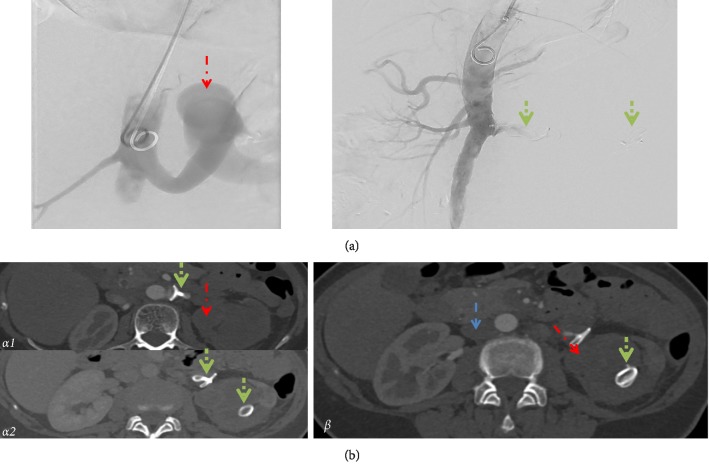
Representative images of an angiography during surgery and a CT scan one-month post-surgery. (a) Angiography before and after Amplatzer Vascular Plug (AVP) II Device placement. No perfusion of the left renal artery (LRA) after AVP II placement. Red arrow (dashed and dotted) = RVA; Green arrow (dotted) = correct positioned central and displaced peripheral AVP II. (b) *α*: arterial phase images. *α*1: no perfusion of the LRA distal of the central AVP II. *α*2: minimal residual cortical perfusion over a side-branch originating from the LRA proximal of the central AVP II. *β*: venous phase image: no contrast in the left vs. right kidney indicating subtotal infarction, despite insignificant residual cortical perfusion. No residual perfusion of the RVA (red arrows, dashed, and dotted) and marked reduction of the IVC diameter (blue arrow, dashed).
